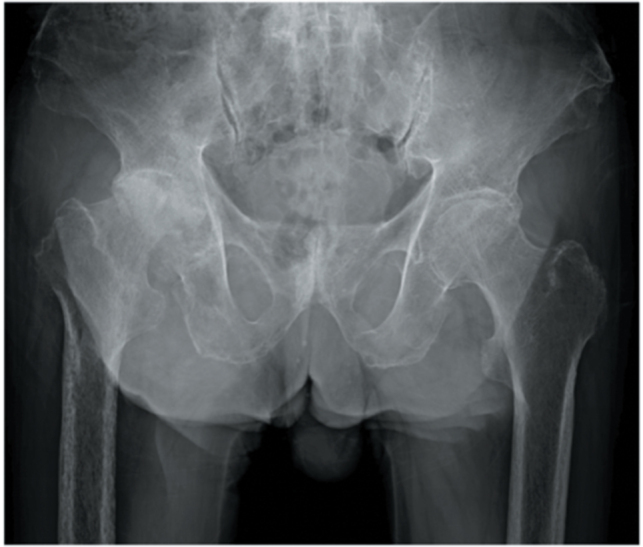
An anteroposterior radiograph of the hip joint after 3 months. The right femoral head is
